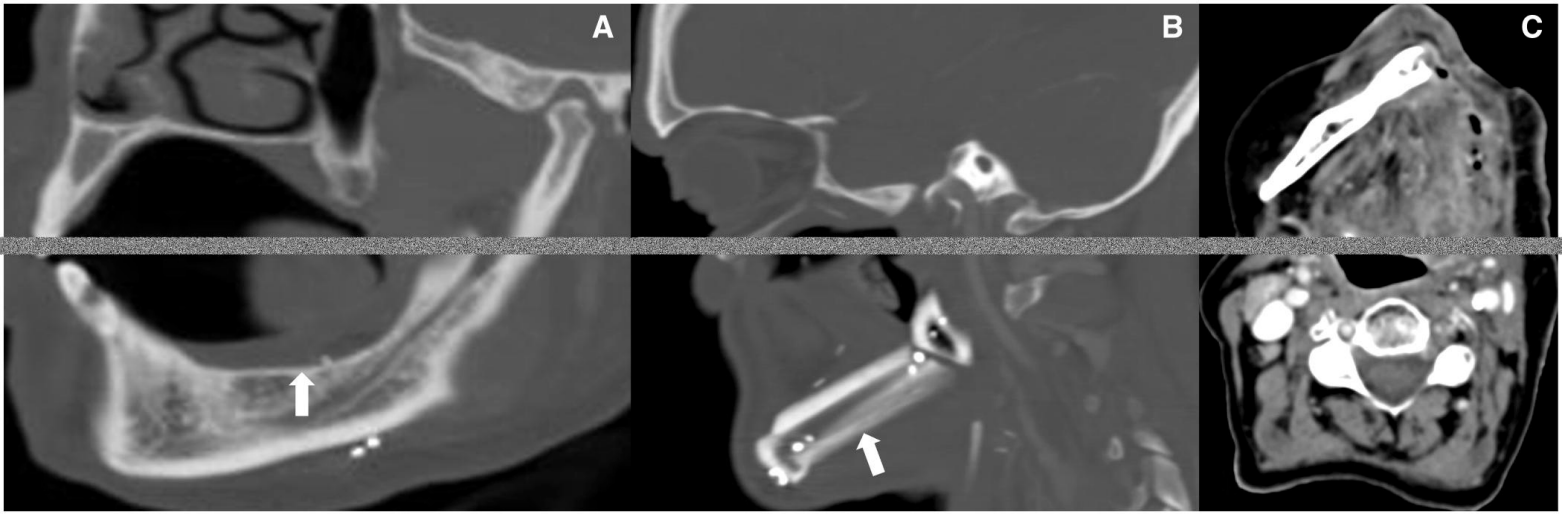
(A) CT with bone algorithm shows post-marginal mandibulectomy status with removal of a rim of mandible including cortex and preserved mandibular continuity and medullary cavity. (B) CT with bone algorithm shows post-segmental mandibulectomy status with removal of a segment of mandible resulting in discontinuity which has been filled with fibular graft (arrow) here (sutures are seen). (C) CECT shows post left hemimandibulectomy status with resection of one half of mandible from midline. In addition, recurrence is seen at the post-operative site.
